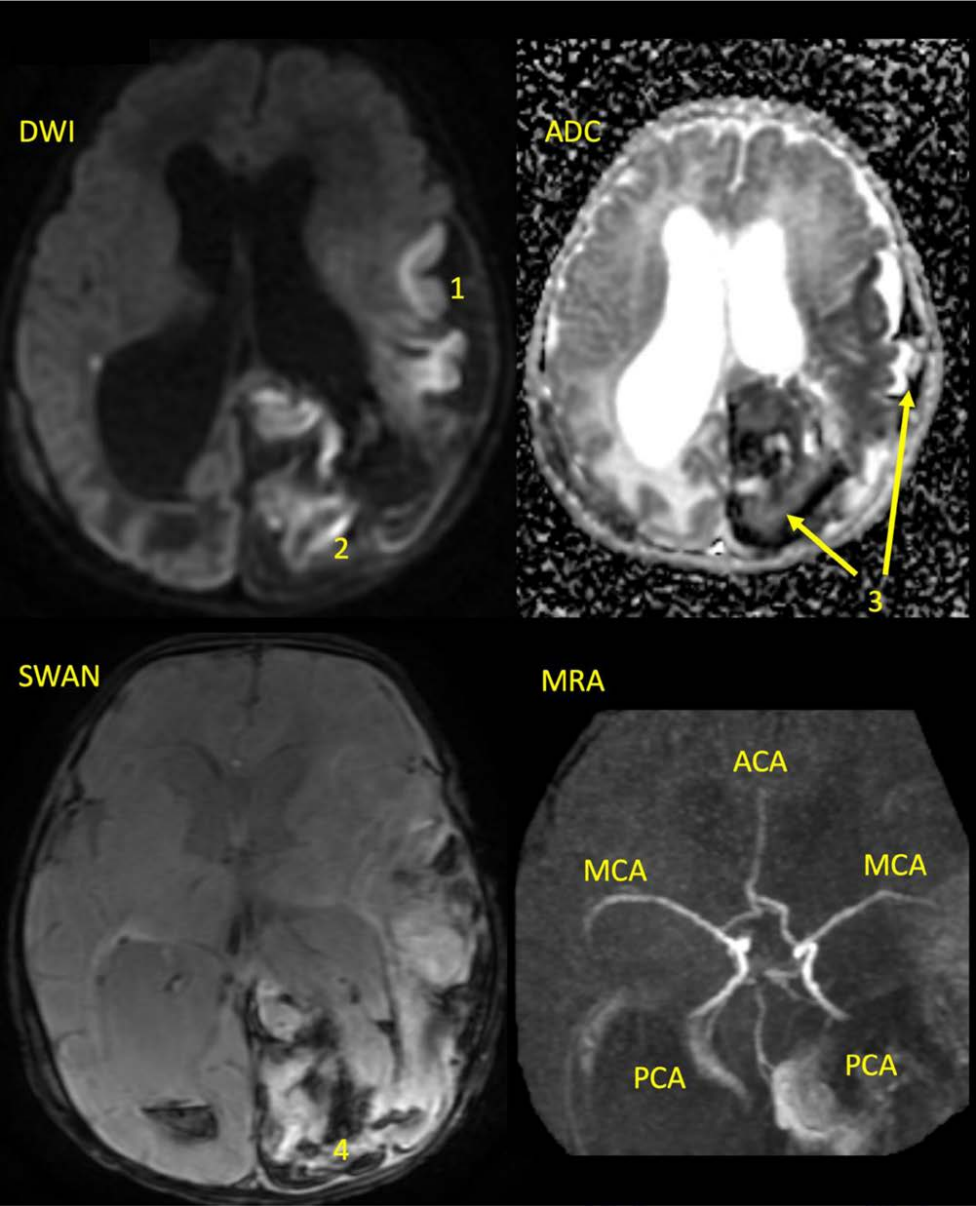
Brain MRI at 6-day-old. DWI: a large area of brain tissue in the left frontal-temporo-parietal lobe (1) and occipital lobe (2) is bright (hyperintense), indicating diffusion restriction. This finding suggested cytotoxic edema due to acute ischemic stroke of the left MCA and PCA. ADC map: the infarction area is dark (hypo intensity) (3) because of the low diffusion coefficient. SWAN: hemorrhage (4) due to acute ischemic stroke of the left PCA territory with hemorrhagic transformation. MRA: no occlusion of left MCA or PCA. ACA = anterior cerebral artery; ADC = apparent diffusion coefficient; DWI = diffusion weighted imaging; MRA = magnetic resonance angiography; MRI = magnetic resonance imaging; PCA = posterior cerebral artery; SWAN = susceptibility-weighted angiography.
